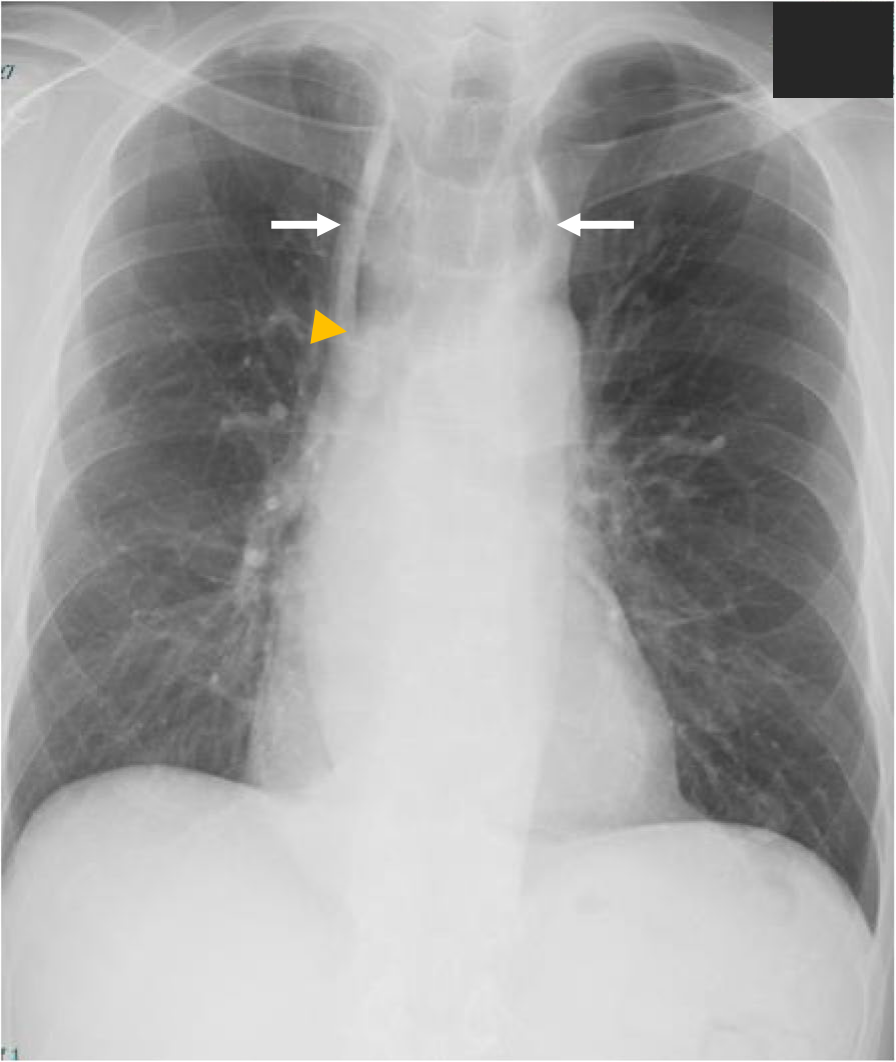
Chest X-ray demonstrated enlargement of the mediastinum (white arrows) and presence of air-fluid levels in the thoracic esophagus (yellow arrowhead)
